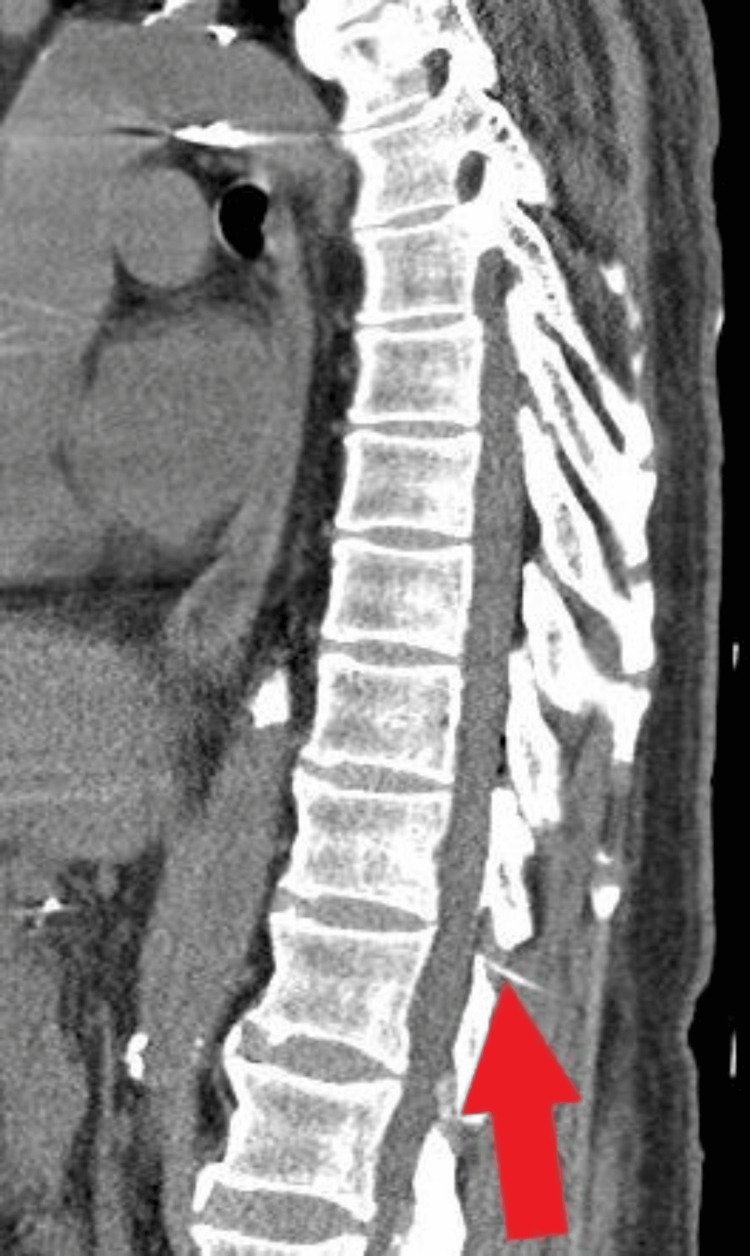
Sagittal CT scan of the thoracic and lumbar spine, with red arrow pointing to the epidural catheter located between the lamina of T11 and T12.
